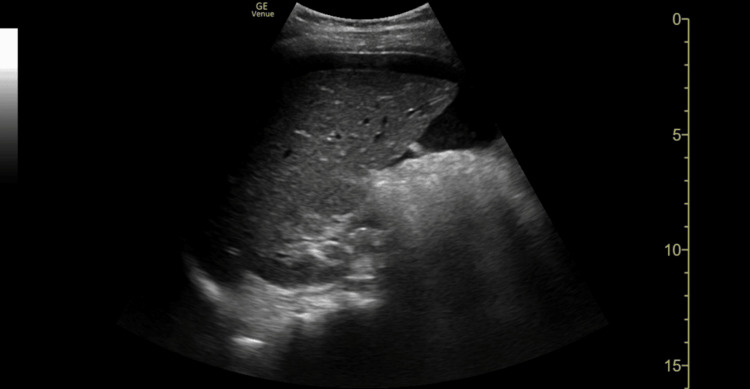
FAST scan of the RUQ demonstrating free intraperitoneal fluid at the caudal tip of the liver FAST: focused abdominal sonography in trauma, RUQ: right upper quadrant
